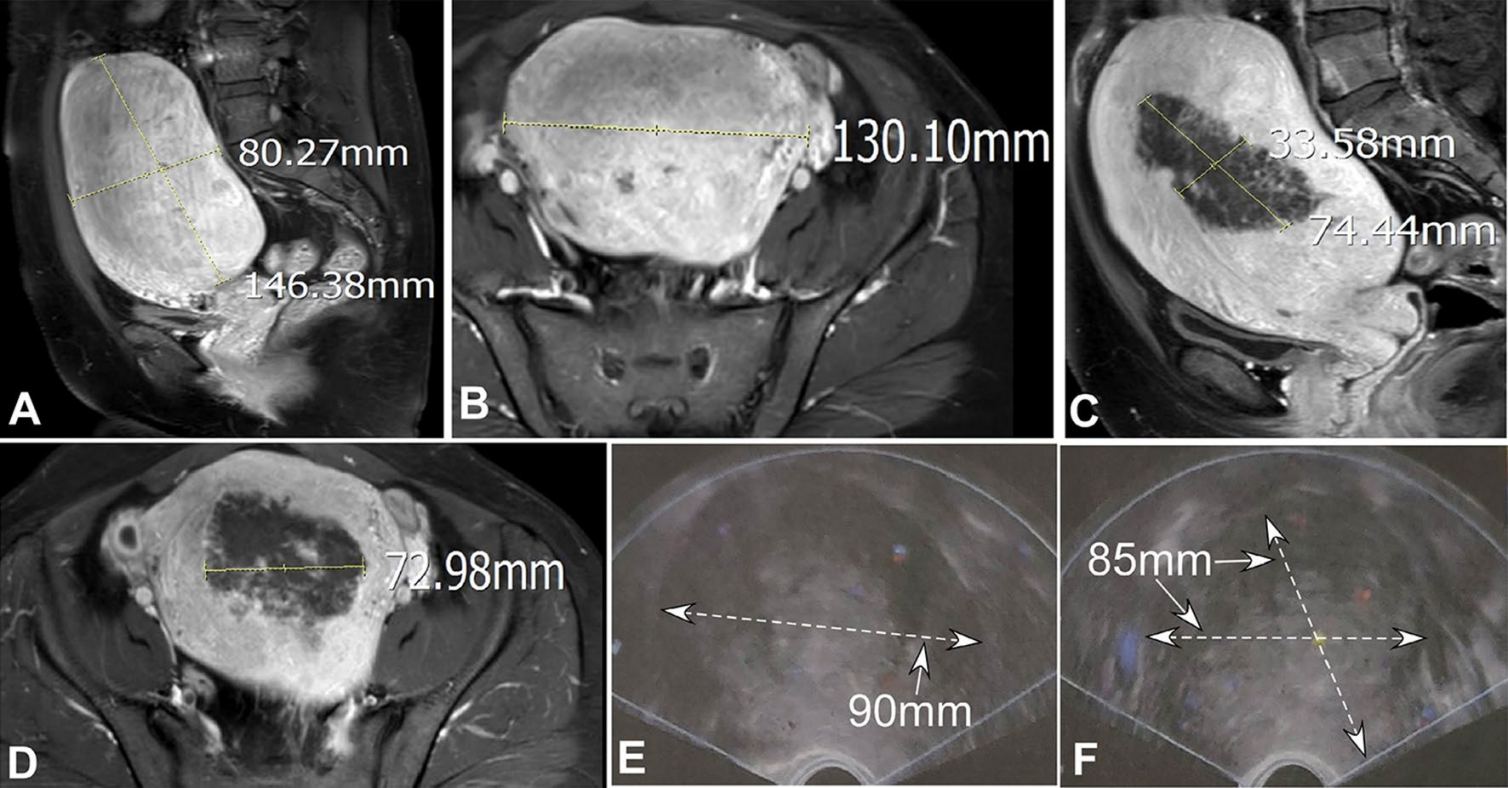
A female patient in their early 40 s with dysmenorrhea and increased menstrual volume for ten years was detected to have uterine adenomyosis. (A) and (B) On enhanced magnetic resonance imaging (MRI), the uterus measured 146.38 mm in the long diameter, 80.27 in the anteroposterior diameter (A) and 130.10 mm in the transverse diameter (B). (C) and (D) After HIFU treatment, the ablative area of the uterus was 74.44 mm in the long diameter, 33.58 mm in the anteroposterior diameter (C), and 72.98 mm in the transverse diameter (D). (E) and (F) Three months after treatment, the uterus was spherical in shape with the size of 85 mm × 9 mm × 85 mm (arrows), the posterior wall of uterus was diffusely thickened, with an unclear boundary, and the blood flow was not rich. The echo in the right fundus was uneven with an unclear boundary is unclear and no blood flow distribution.
